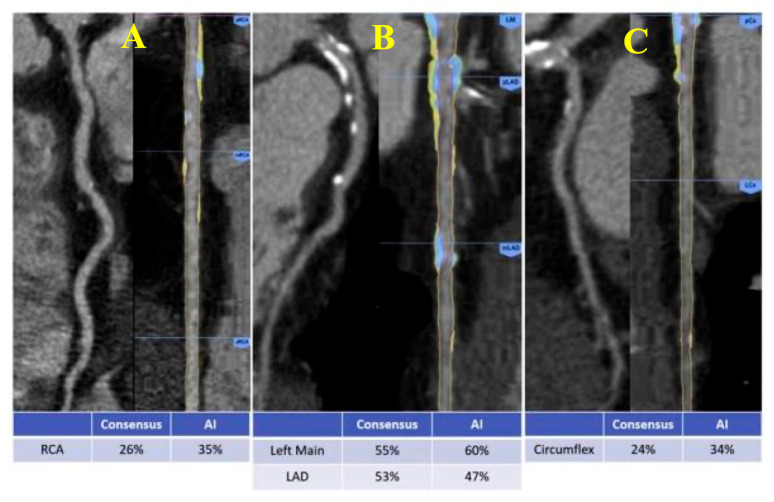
Automation in Coronary Computed Tomography Angiography (CCTA). Comparison of stenosis assessment performed by an expert Level-3 reader versus AI-based automated analysis across major coronary arteries: (A) Right coronary artery (RCA); (B) Left main (LM) and Left Anterior Descending Artery (LAD); (C) Left Circumflex Artery (LCx). Adapted from Choi AD et al. [33].
